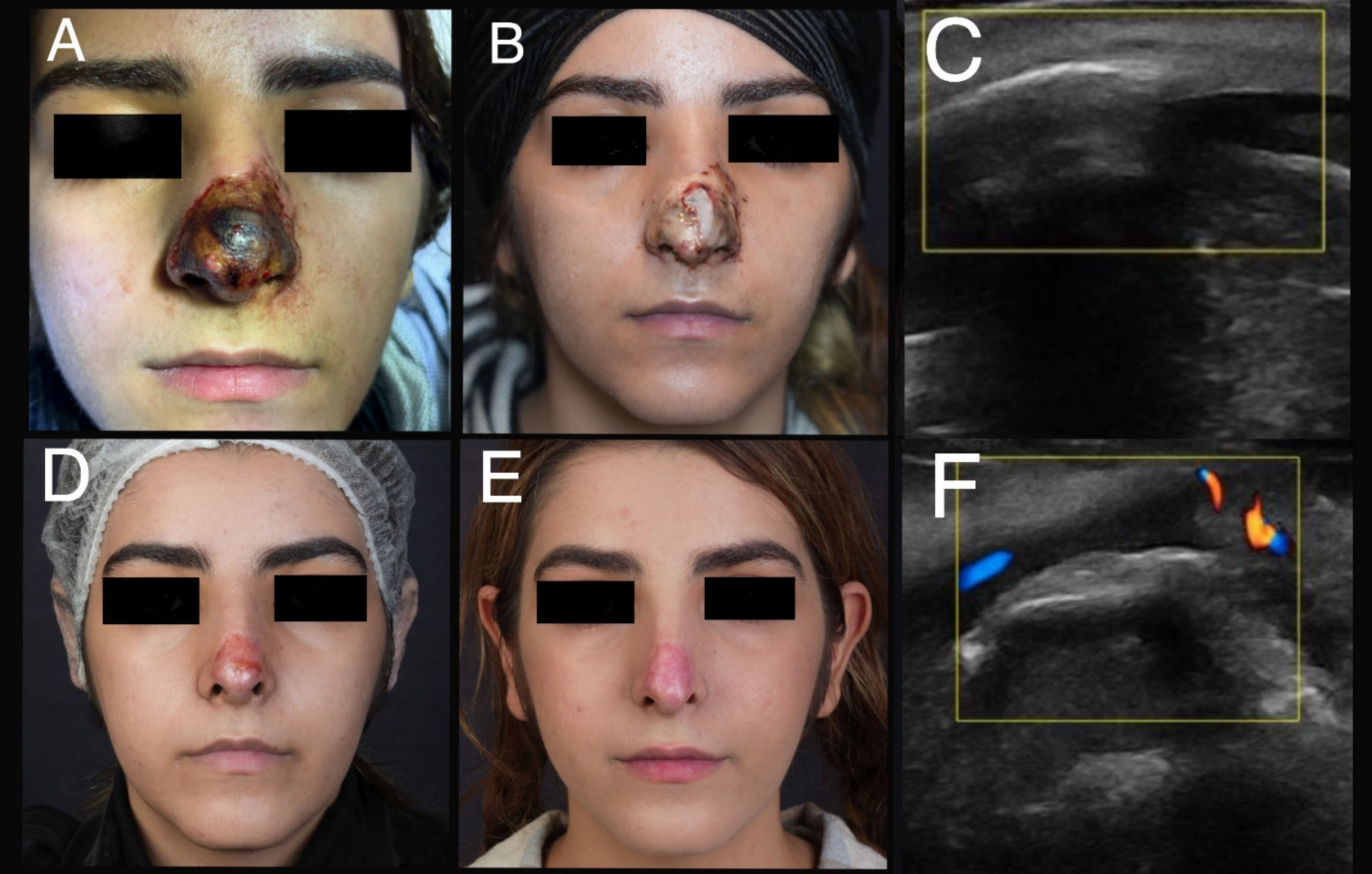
A 17-year-old female experienced stage five of ischemia following an injection of 1 cc HA filler. (A) A clinical presentation after 144 h of filler injection. (B) A day after treatment with “THIS and FAT” protocol. (C) An ultrasound examination before treatment (Involvement of lateral nasal artery). (D) Improvement of the lesion after 3 months. (E) Atrophic scar after 8 months of filler injection. (F) An ultrasound examination after treatment (arterial flow return to normal).
